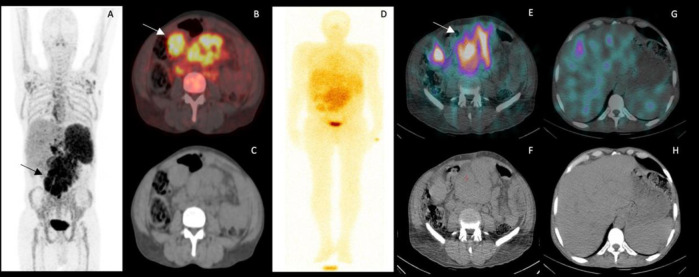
A 52yr/Male, c/o RR-MCL, post 6 cycles of R-CHOP underwent ^68^Ga-Cyclic pentapeptide (A-C) and ^177^Lu-Cyclic pentapeptide (D-H) imaging. MIP image of ^68^Ga- Cyclic pentapeptide (A) showed tracer uptake in the mesenteric mass and corresponding fused PET/CT (B) and CT images (C) of the abdomen showed high tracer avidity in the mesenteric mass at L4 level (SUV_max_=22.4, arrow). Whole-body planar images were acquired post intravenous administration of 473.6 MBq^177^Lu-Cyclic pentapeptide in the same patient up to 3 days for dosimetry purpose (Image D, Day 1). Axial fused SPECT/CT and corresponding CT images of the abdomen (E & F), showed high ^177^Lu-Cyclic pentapeptide avidity in the mesenteric mass (arrow). Physiological uptake of ^177^Lu-Cyclic pentapeptide was noted in the liver, spleen, kidneys, and high blood pool clearance. SPECT/CT image (E) was acquired at 24 h post radiotracer injection. In addition, faster clearance from hepatic tissues was noted at 24 h in the fused SPECT/CT imaging (G) and corresponding CT images (H)
